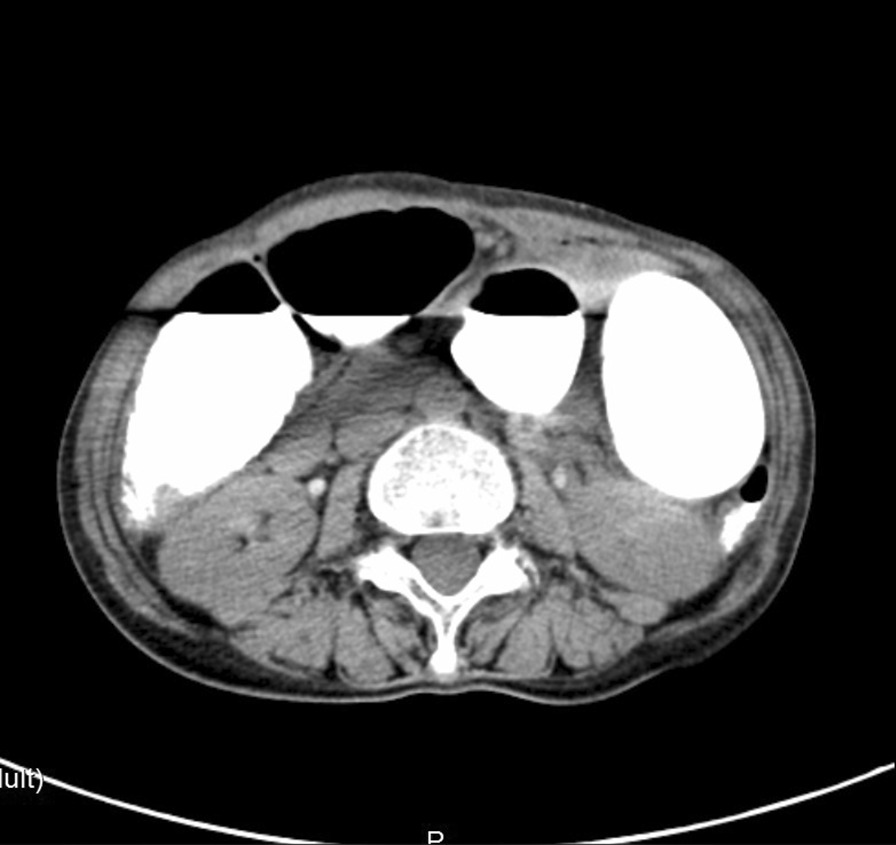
Reexamination of abdominal CT showed that the intestinal obstruction was not significantly relieved
